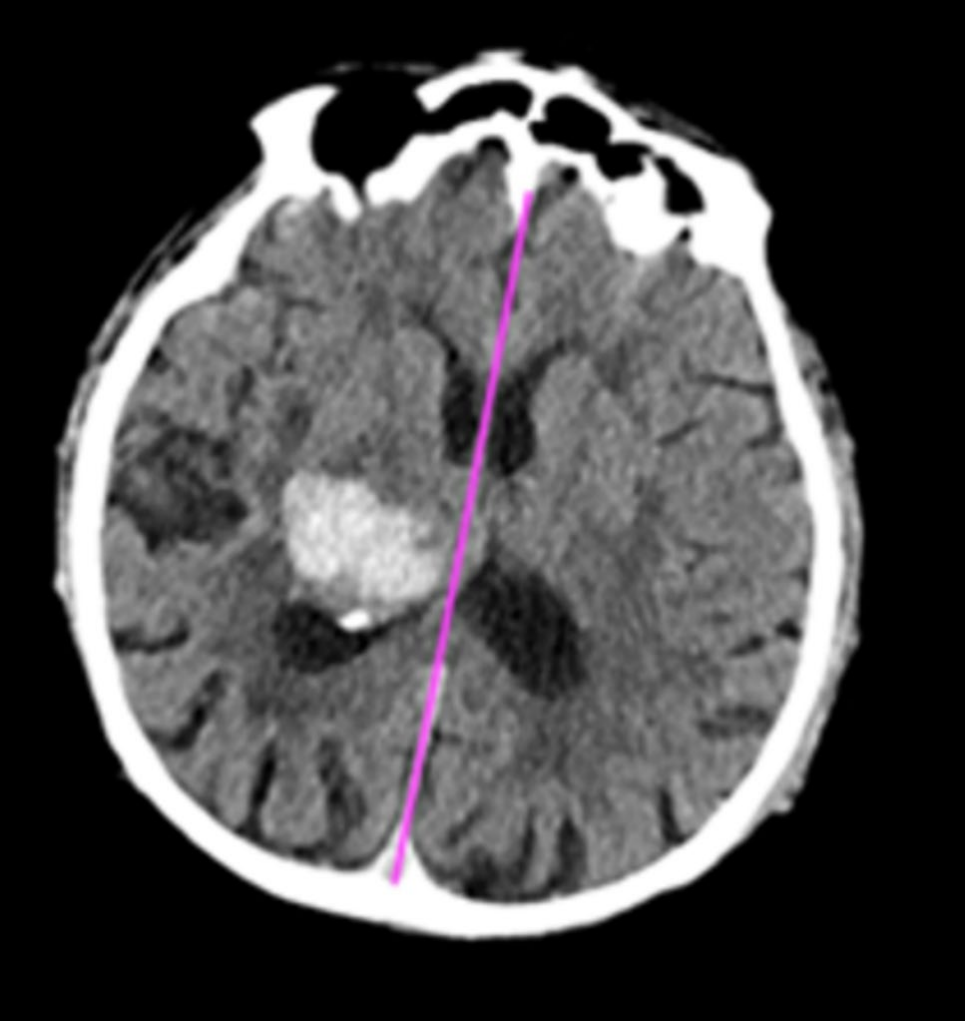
CT scan with the presence of a basal ganglia hemorrhage with related midline shift
